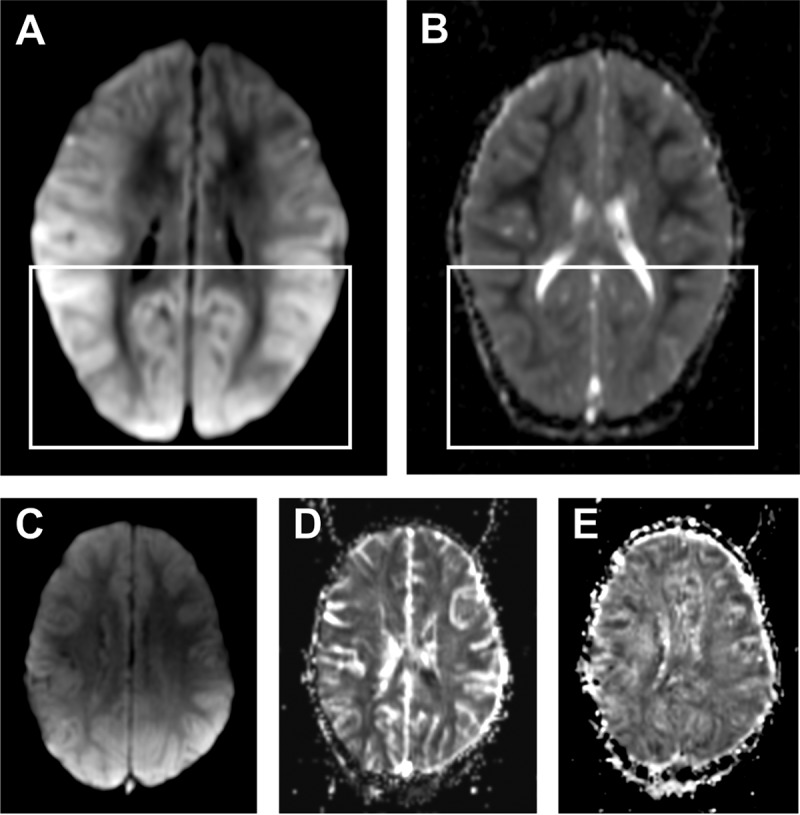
Case ID41. Increased DWI signal in the cortex on admission, with posterior predominance (A) and no mismatch in corresponding ADC image (B), consistent with vasogenic edema (white boxes). Cortical increase of CBF (C) and CBV (D) with minimal reduction of MTT (E) in the same area.
